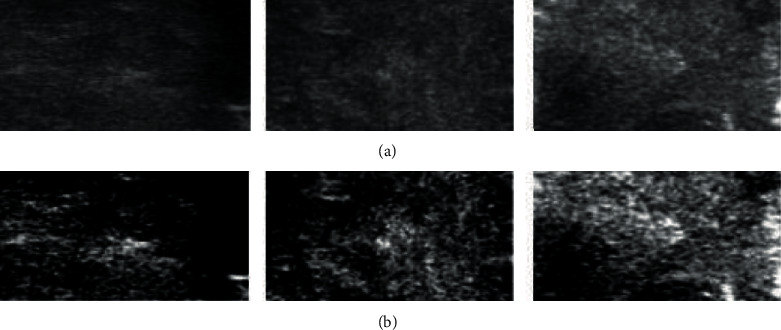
(a) Transrectal ultrasound prostate image and (b) optical density image.
